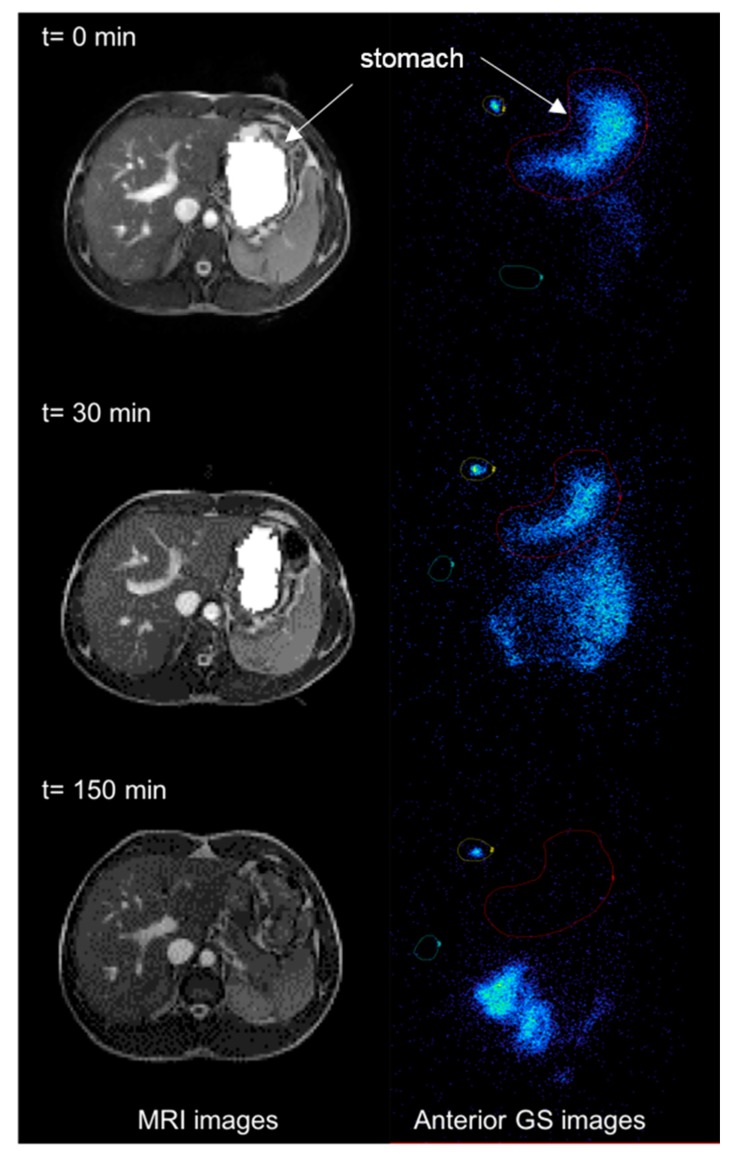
Example of magnetic resonance imaging (MRI) and Gamma scintigraphy (GS) images of the stomach at different time points after a participant ate the soup test meal (t = 0 immediately after feeding, t = 30 min after feeding and t = 150 min at the end of the study period). The axial, moderately T2 weighted images are shown on the left hand of the panel and the coronal planar gamma scintigraphy images are shown on the right hand of the panel, with labels and arrows to indicate anatomical landmarks. Regions of interests (ROIs) were defined around the labelled meal in the stomach (red), around an area of background activity (green) and around the radioactive marker (yellow).
